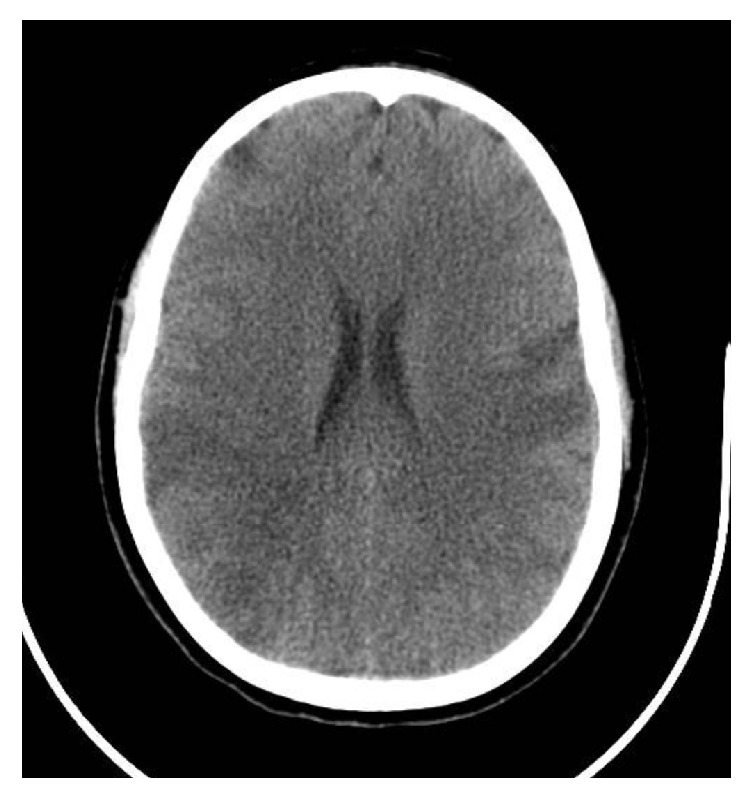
Image from the patient's CT brain without contrast demonstrating multiple acute infarcts, including right middle cerebral artery (MCA) territory.
